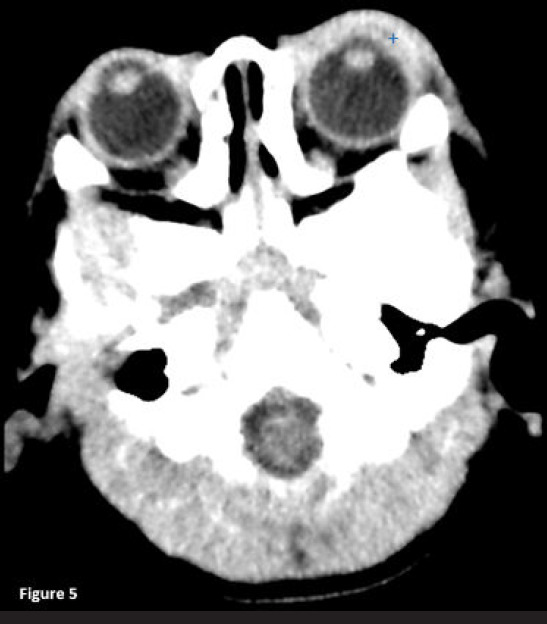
Computed tomography scan of the brain and orbit showing left periorbital soft tissue swelling with no fat stranding [+] representing left periorbital cellulitis.
